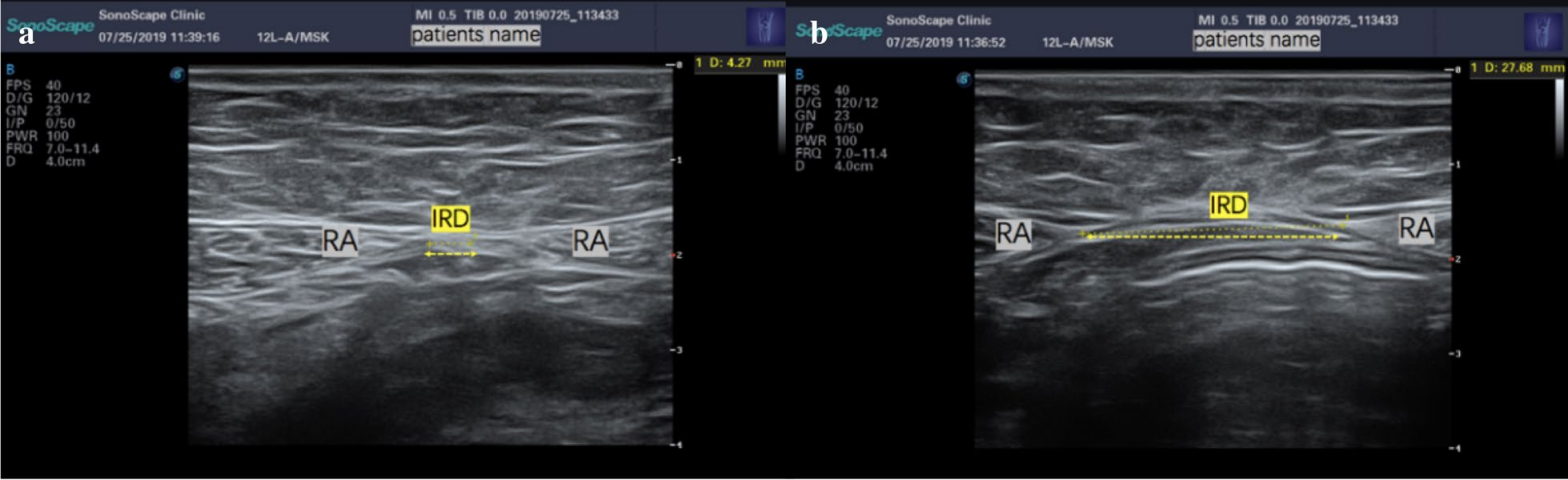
Ultrasound images. a Shows a normal situation for inter-rectus distance (< 20 mm); b Shows a situation of diastasis of rectus abdominis (≥ 20 mm); the subjects’ name are covered for safeguard subjects’ privacy. IRD, inter-rectus distance; RA, recti abdominis
